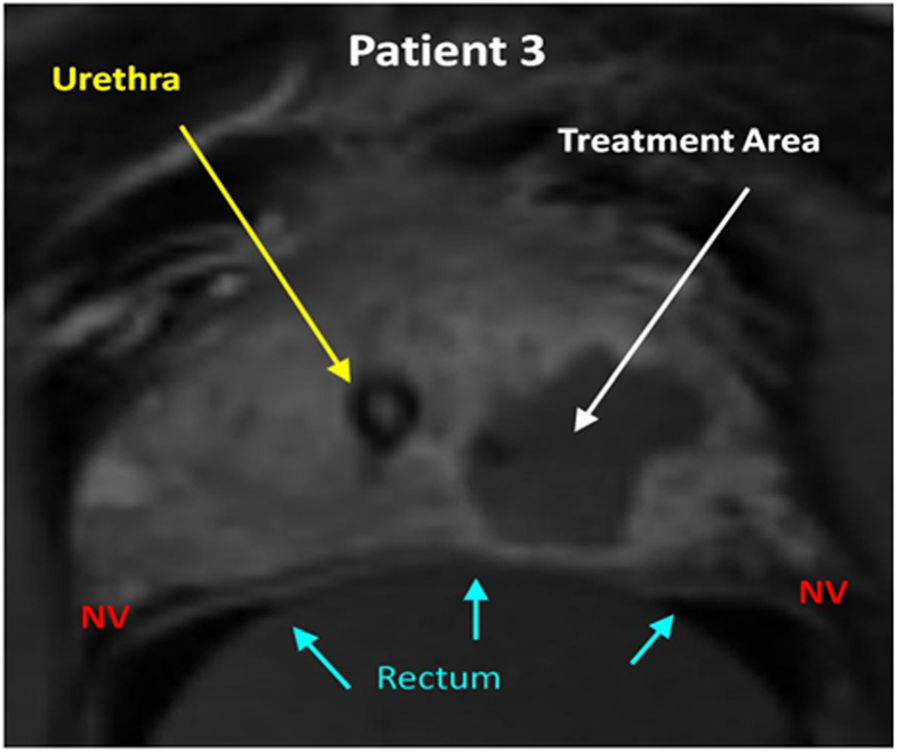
Post contrast T1-weighted axial image immediately after the completion of therapy in patient 3 with Gleason 6 disease in the left anterior medial apex, left posterior lateral apex, left posterior medial apex, and left posterior medial base. The treatment area demonstrates non-perfusion of tissue after MRGFUS thermal ablation. *NV* neurovascular bundle
